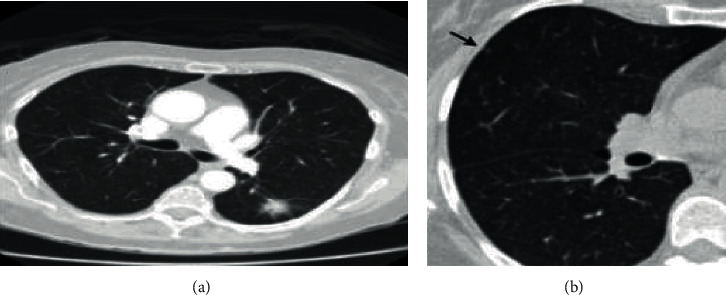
(a) Lung cancer nodule view. (b) An arrow indicates this 1.25 mm thick CT slice to contain approximately 2 mm long lung nodules.
